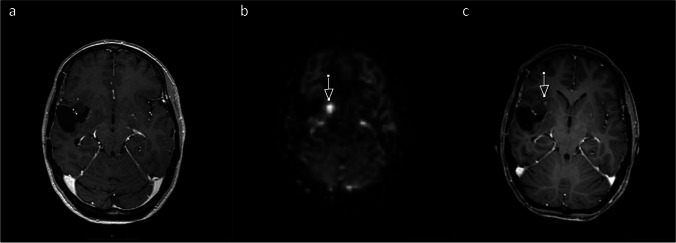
Forty-three-year-old woman with an untreated oligodendroglioma. True positive scan; 4.a Index T1-MRI with contrast agent: no contrast enhancement. 4.b Index ASL rated as positive. 4.c Follow-up T1-MRI with contrast agent (17 days after index MRI): new contrast enhancement. All axial planes
